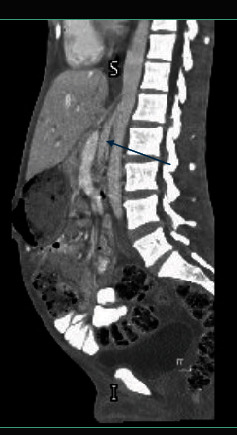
CT scan sagittal reconstruction demonstrates a narrow aortomesenteric with compressed left renal vein.
